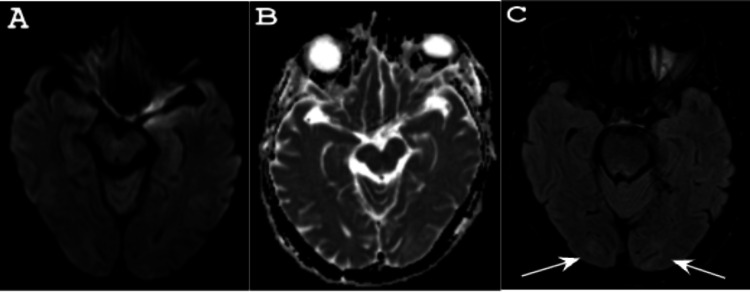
MRI of the brain post-treatment (A, B) Axial DWI and ADC again showing no evidence of diffusion restriction, while (C) FLAIR shows improvement of the bilateral hyperintensities in the right parietal and bilateral occipital lobes following improvement in PaCO₂ levels after six days. MRI: magnetic resonance imaging; DWI: diffusion-weighted imaging; ADC: apparent diffusion coefficient; FLAIR: fluid-attenuated inversion recovery; PaCO₂: arterial partial pressure of carbon dioxide
